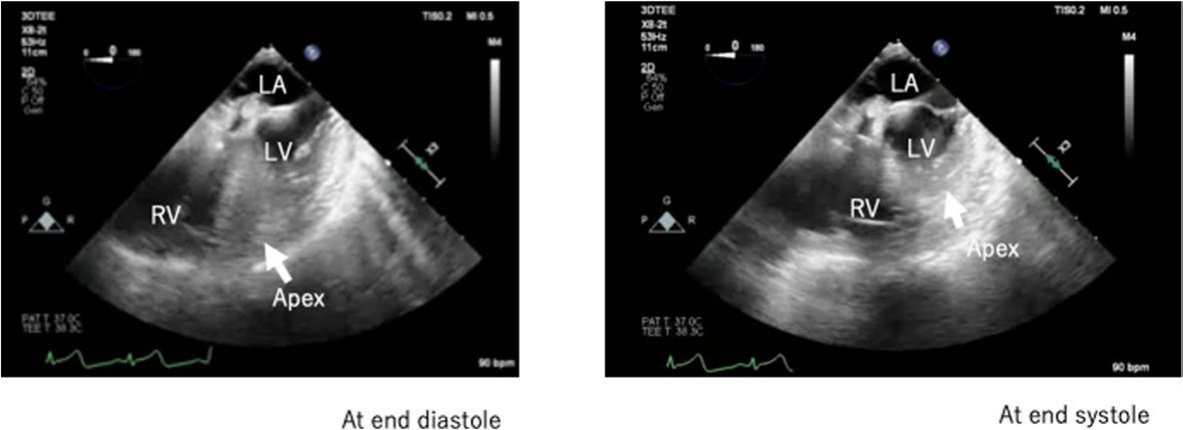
Four-chamber mid-esophageal echocardiographic images at end diastole (left) and end systole (right). The left ventricular apex shifted significantly during the cardiac cycle
